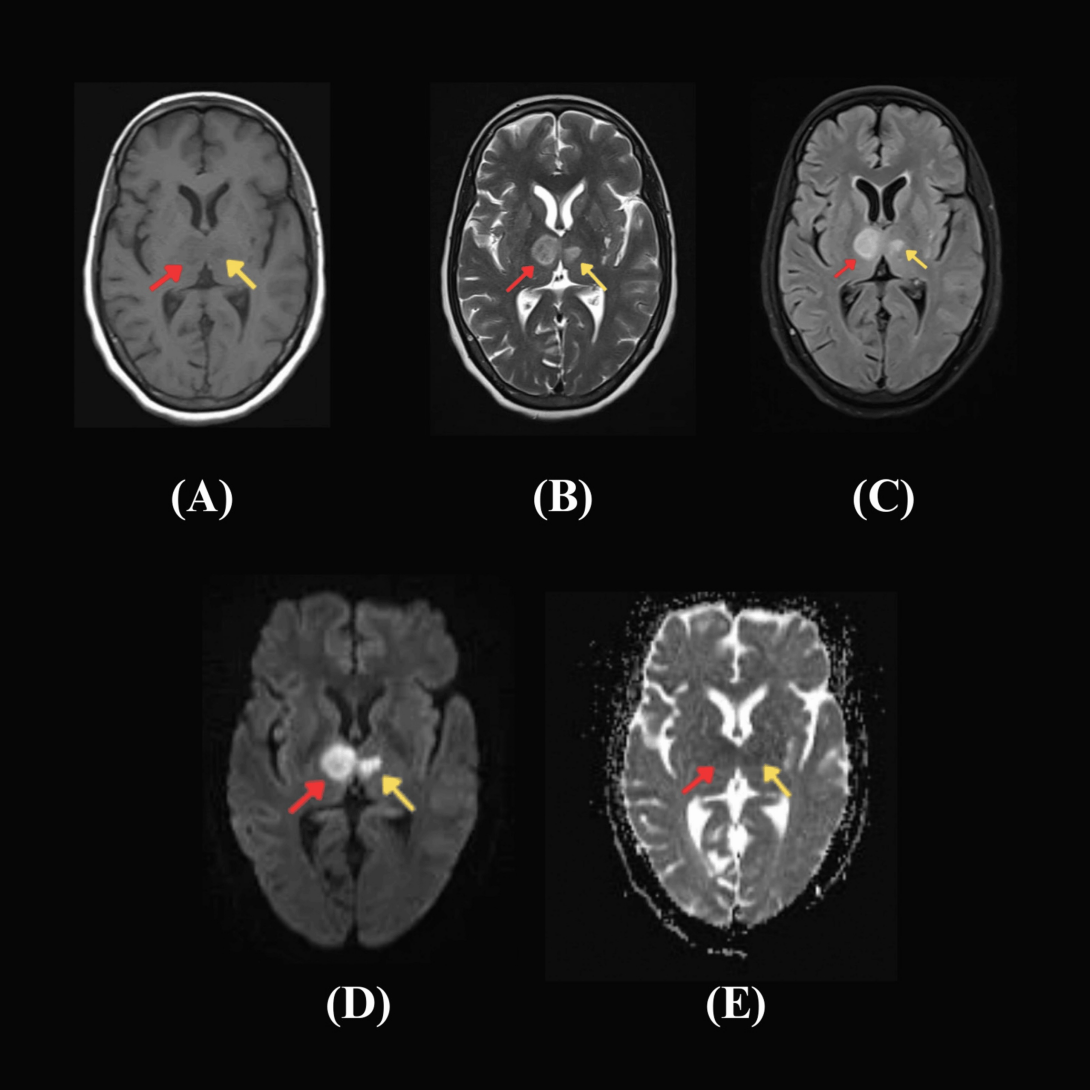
Magnetic resonance imaging (MRI) of the brain MRI findings: (A) - Axial T1: Hypointense signals in both thalami, more on the right. (B)/(C) - Transverse T2/FLAIR: Hyperintense signals in bilateral thalamus, predominantly right, extending to midbrain. (D) and (E) - Diffusion-weighted imaging and Asymmetric diffusion coefficient map: Asymmetric diffusion restriction in bilateral thalamus, primarily on the right side expanding to bilateral midbrain paramedian to midline. Right thalamus, red arrow; left thalamus, yellow arrow. Focal mass effect in the form of mild effacement of the third ventricle – all these features suggestive of acute infarct. FLAIR, fluid-attenuated inversion recovery.
